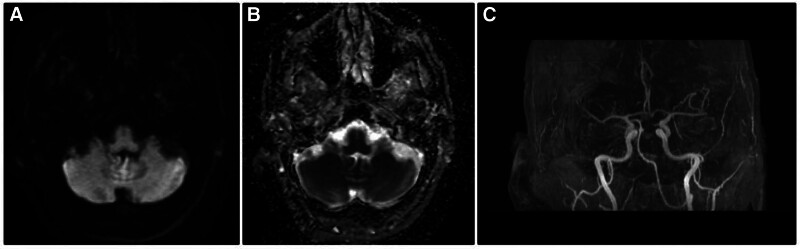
(A) Diffusion weighted imaging (DWI) showing no acute ischemia, (B) confirmed with apparent diffusion coefficient (ADC), (C) magnetic resonance angiography (MRA) showing no occlusion or narrowing. ADC = apparent diffusion coefficient, DWI = diffusion weighted imaging, MRA = magnetic resonance angiography.
